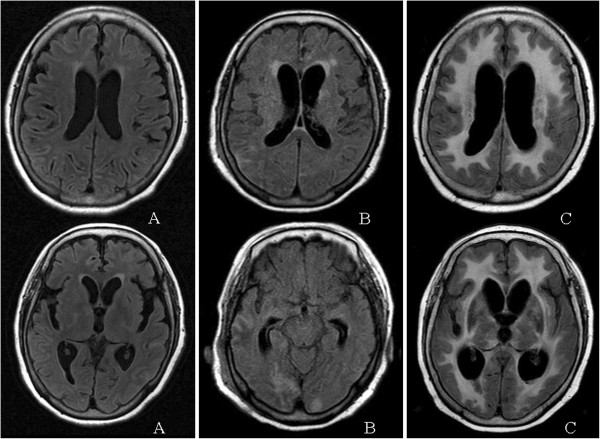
Axial fluid-attenuated inversion recovery (FLAIR) brain MRI scans of the patient with CAA-ri. (A) At time of admission, there was increased signal intensity in the leptomeninges and sulci; (B) At 49 days after admission, there were more extensive hyperintensity lesions in the leptomeninges and sulci, and there was asymmetric subcortical white matter of the occipital lobes; (C) At 195 days after admission, the hyperintensity lesions extended diffusely through the white matter.
